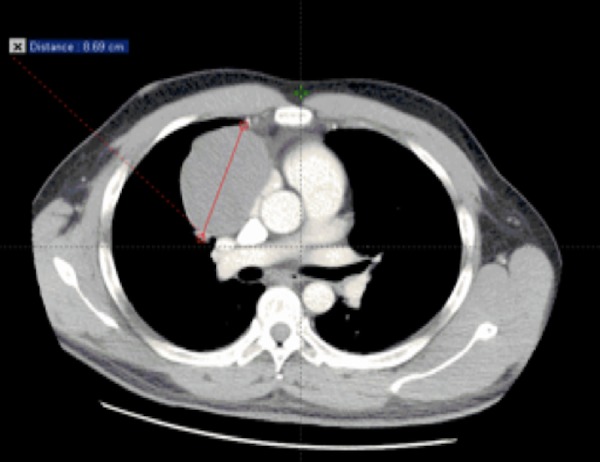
Axial contrast-enhanced computed tomography scan of thorax showing the right anterior mediastinal mass.
